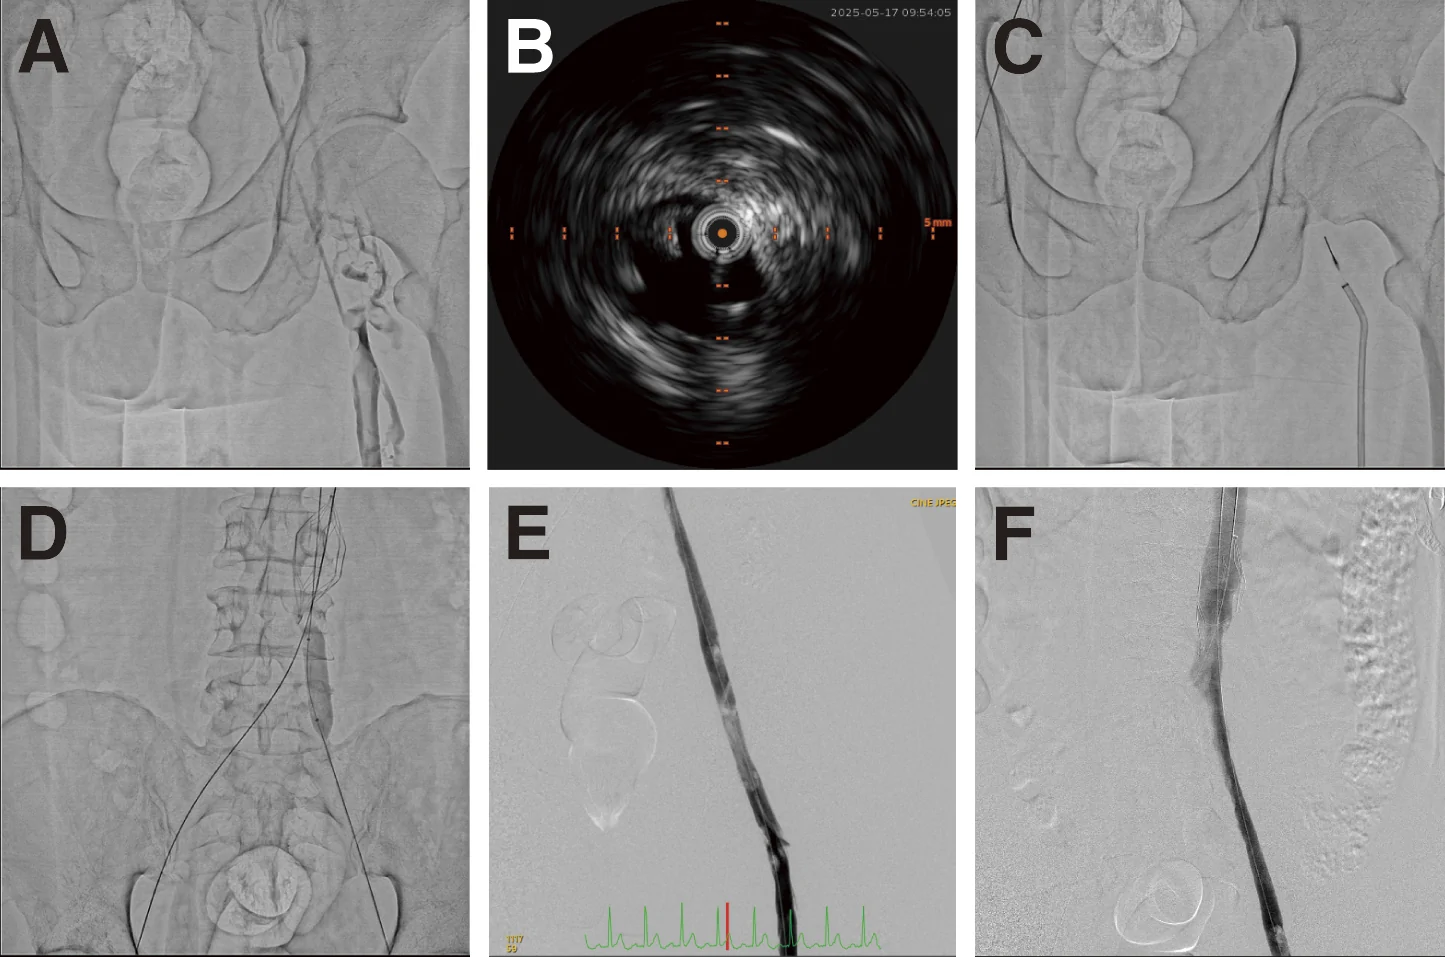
A venography shows a large amount of residual thrombus in the right leg vein, though slight antegrade flow is restored (A). IVUS reveals thrombus below the IVCF in the IVC disappeared (B). The residual thrombus in the right leg is aspirated with a CAT8 catheter (C). The right iliac vein and common femoral vein are dilated with a balloon with a 12-mm diameter (D). The final venography shows restoration of antegrade blood flow, with a little residual thrombus (E, F). Because of the prone position, each venography shows the right leg vein. IVUS, intravascular ultrasound; IVC, inferior vena cava; IVCF, inferior vena cava filter
